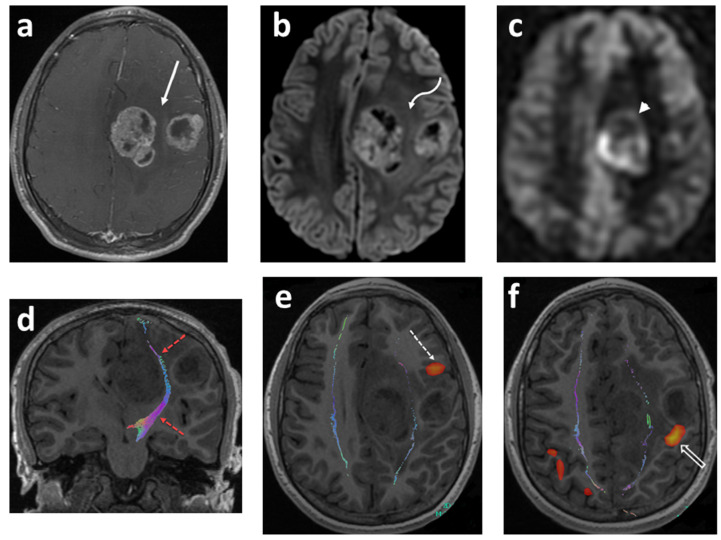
Fourteen-year-old boy with headaches and episodes of right facial and hand numbness. Axial T1 fat sat post contrast (a); axial DWI (b); axial ASL-PWI (c); coronal T1 tractography (d); and functional (e,f) images. There are two well-defined lobulated heterogeneously enhancing lesions within the posterior aspect of the left frontal lobe (solid white arrow). The lesions show restricted diffusion (curved white arrow) and increased perfusion (arrowhead). The cortical spinal tracts are identified tracking in between the two tumor masses (red–dashed arrows). The Broca’s area is identified in the left inferior frontal gyrus anterior and inferior to the lateral frontal mass lesion (dashed arrow). The motor cortex with right finger tapping is immediately posterior to the smaller mass in the lateral aspect of the left frontal lobe (open arrow). Pathology: giant cell glioblastoma (author’s institutional human ethics committee/institutional review board guidelines were followed for anonymized images).
